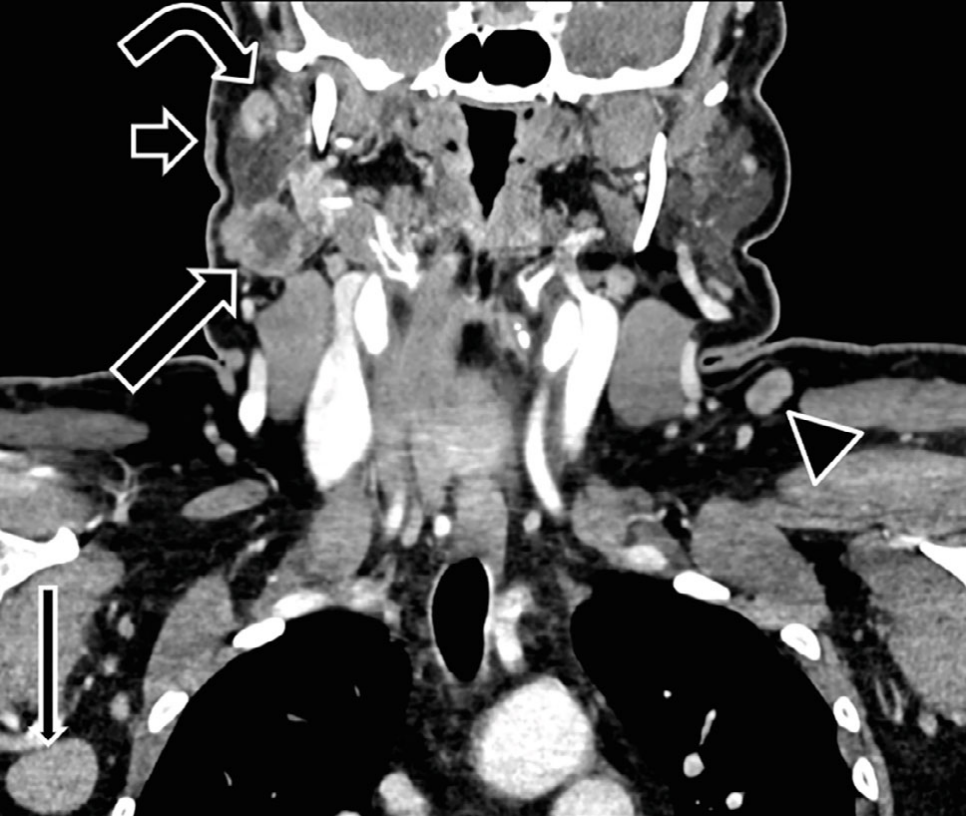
A 66‐year‐old female with a history of basal cell carcinoma presented with a right parotid region lump. Coronal contrast‐enhanced soft tissue neck CT shows a centrally necrotic right parotid tail lesion (long arrow). An enlarged superficial parotid lymph node is present superiorly (curved arrow). Careful inspection reveals skin thickening overlying the parotid, which was the site of the patient′s prior basal cell carcinoma resection (short arrow). Enlarged, rounded nonnecrotic nodes are present in left Level V (arrowhead) and the right axilla (thin long arrow), which is not a typical pattern for disease spread from a right preauricular skin primary. Right parotidectomy showed metastatic basal cell carcinoma and follicular lymphoma, and nodes from right neck dissection showed follicular lymphoma. Notably, the lymphoma was a new diagnosis for this patient.
